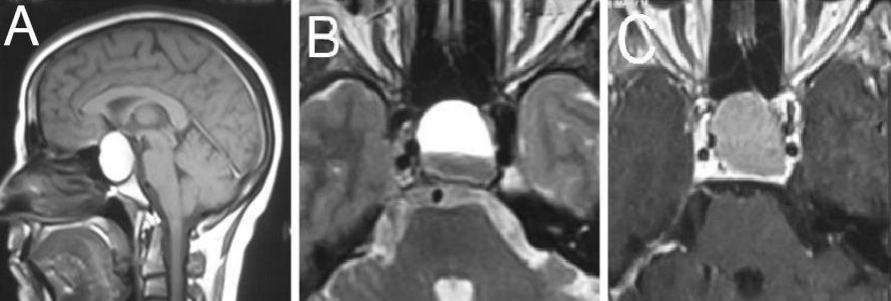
A- In sagittal T2's view, a big cystic tumor was seen in sella turcica that was extended to the suprasellar region; B- In coronal T2's view, it can be seen that optic chiasm was displaced and right optic nerve was under pressure by this cystic mass; C- MRI with gadolinium showed a faint enhancement around the tumor
